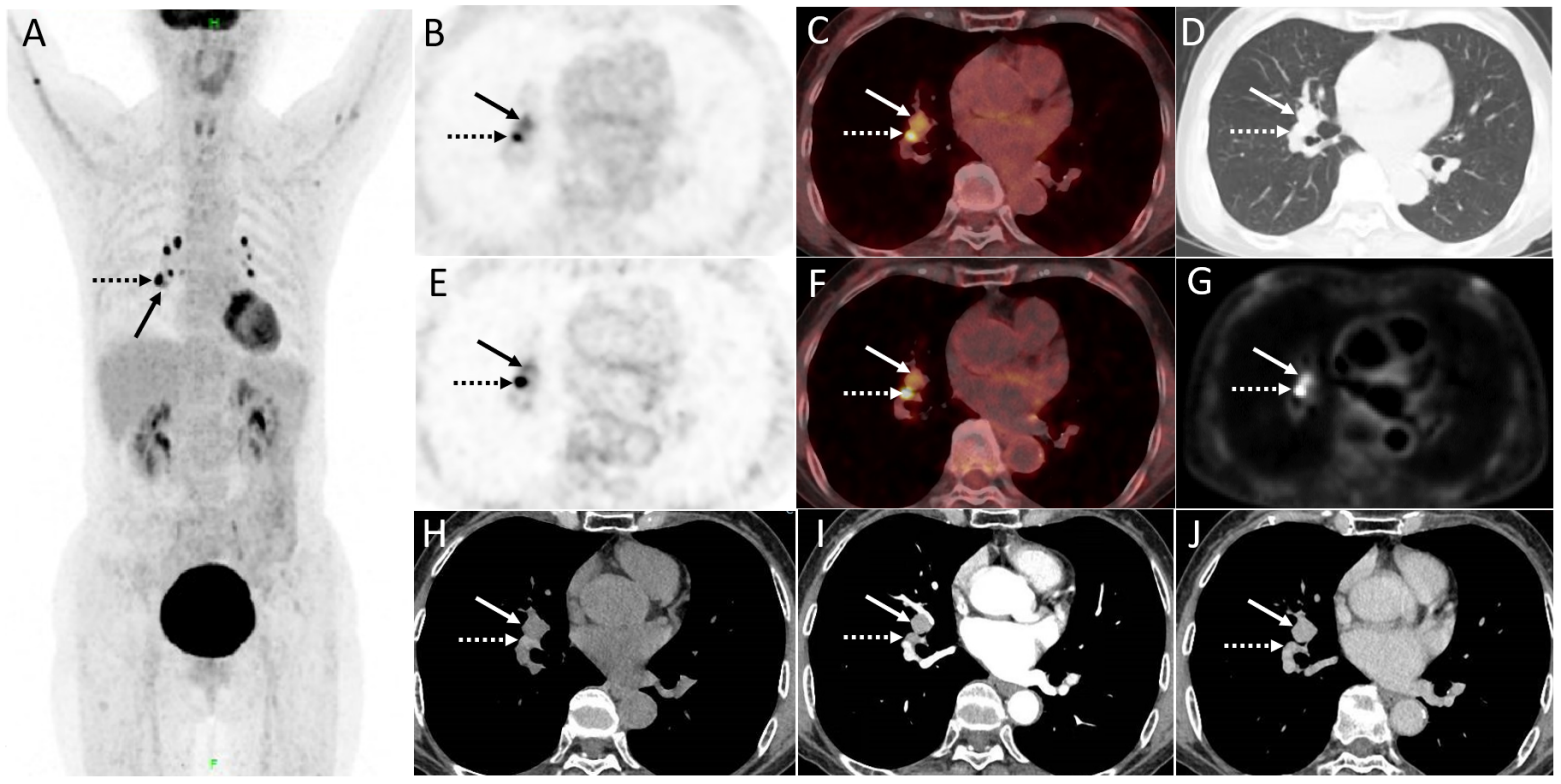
(A) The maximum intensity projection image (MIP) of PET/CT. The described right hilar mass (1.6 cm × 1.5 cm) displayed in (B, C) regular scan, (D) lung window, and (E, F) delay scan (arrow, SUVmax of 3.9 and 3.8, respectively) mimicking mediastinal lymph nodes with intense FDG uptake (dotted arrow, SUVmax 12.3 and 17.4. respectively). (G) Quantification analysis of dynamic parameters (Ki, net influx rate) shows that the mediastinal lymph nodes had a significantly high uptake of FDG but a relatively low Ki value (average Ki of 0.021 mL/g/min), while the Ki value for endobronchial mass (arrow) was 0.029 mL/g/min. (H–J) Contrast-enhanced CT revealed a markedly enhanced endobronchial mass adapting to the right intermediate bronchus.
